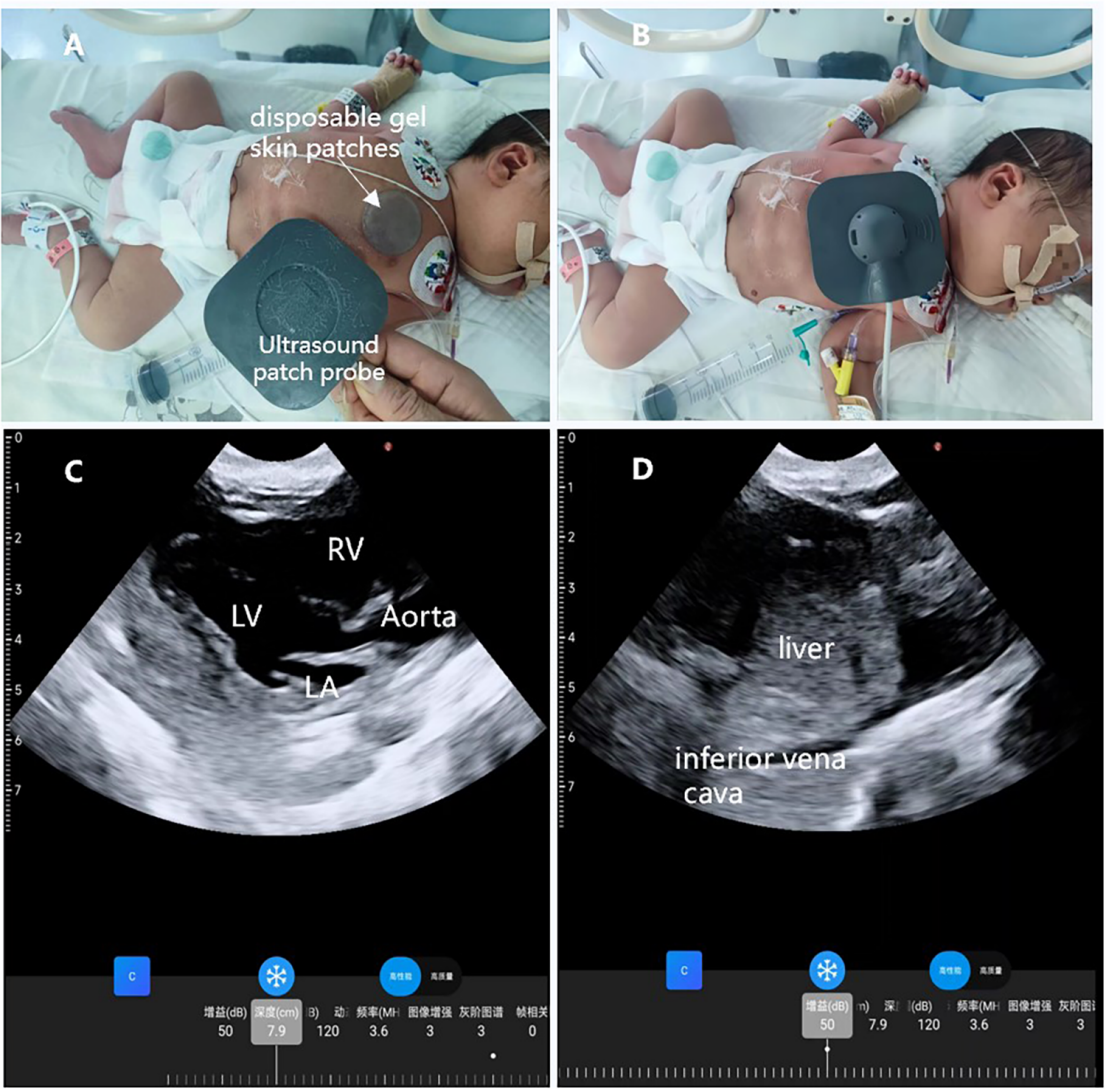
Wearable ultrasound technology is utilized in the NICU. (A) A disposable gel skin patches was placed on the neonate's chest for the ultrasound; (B) the wearable ultrasound patch was placed on the left anterior chest of the neonate; (C) parasternal long-axis view; (D) subcostal view of the inferior vena cava.
